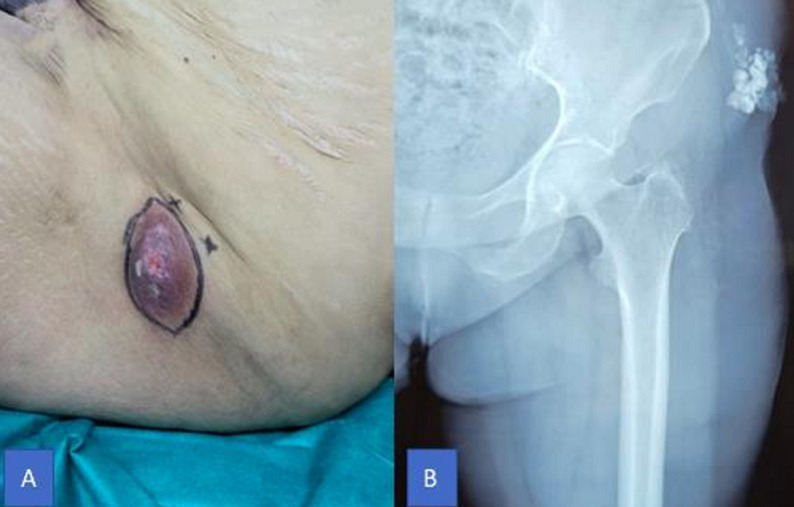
A) masse érythémateuse polylobée dure à la palpation évoluant depuis 2 mois; B) la radiographie standard de face
